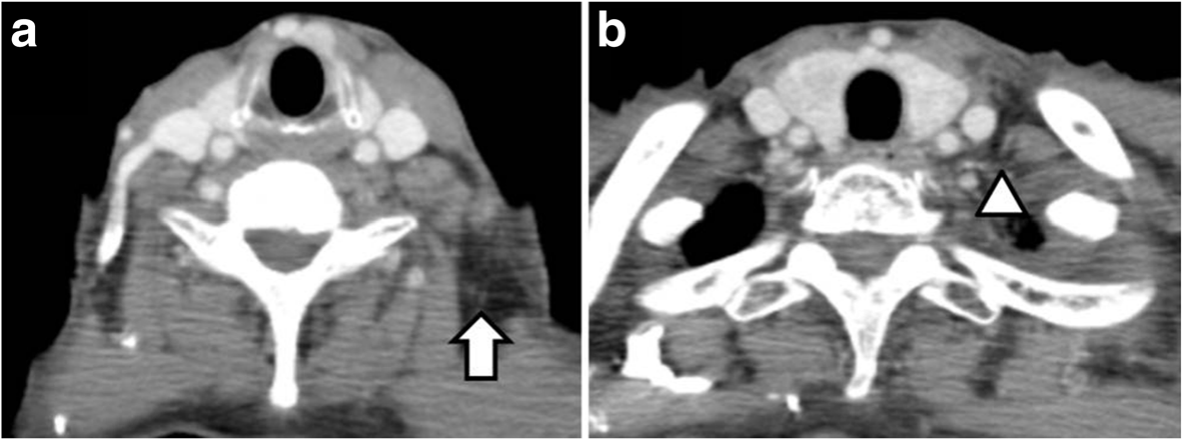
CT after chemoradiation therapy showing complete response to the treatment (a, b)
